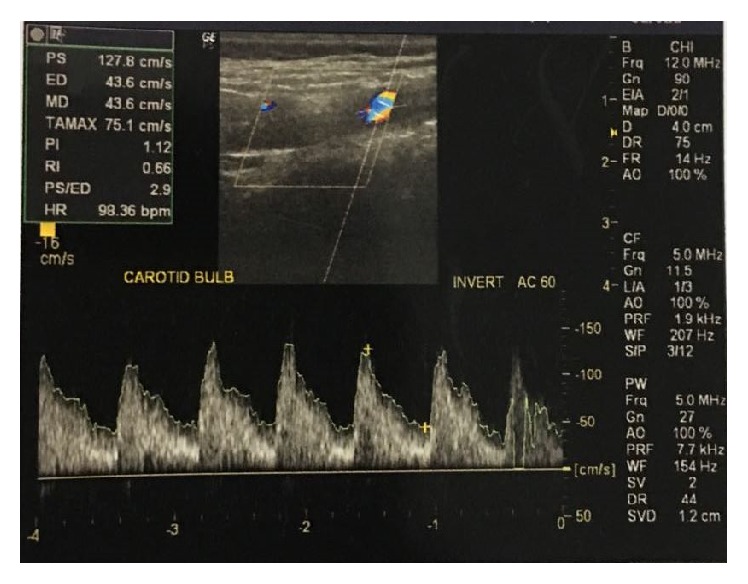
Carotid artery Doppler of the patient.
